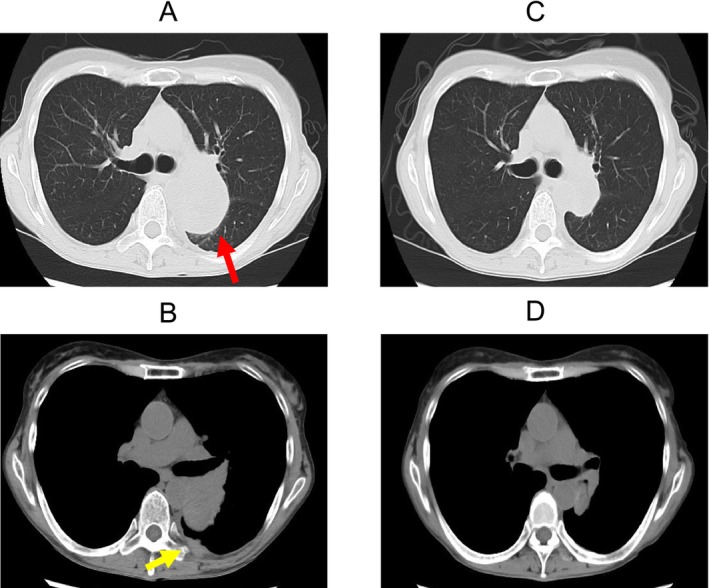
CT images showing a 63 × 42 mm tumour in the left lung (red arrow, A) and pleural metastasis (yellow arrow, B) prior to treatment. After 52 days of treatment, the tumour size reduced to 33 × 24 mm (C), and no pleural nodules were observed (D).
